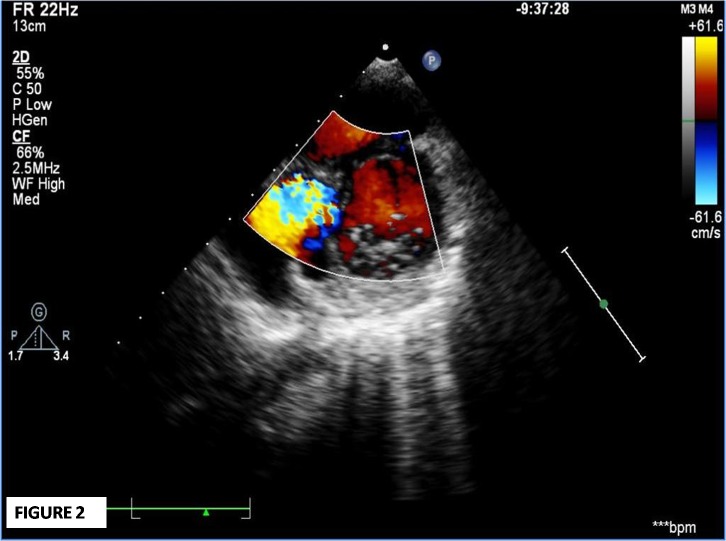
Figure 2:Parasternal short axis view at mitral valve level demonstrates communication between aneurysmal sac and left ventricular cavity on color flow.
